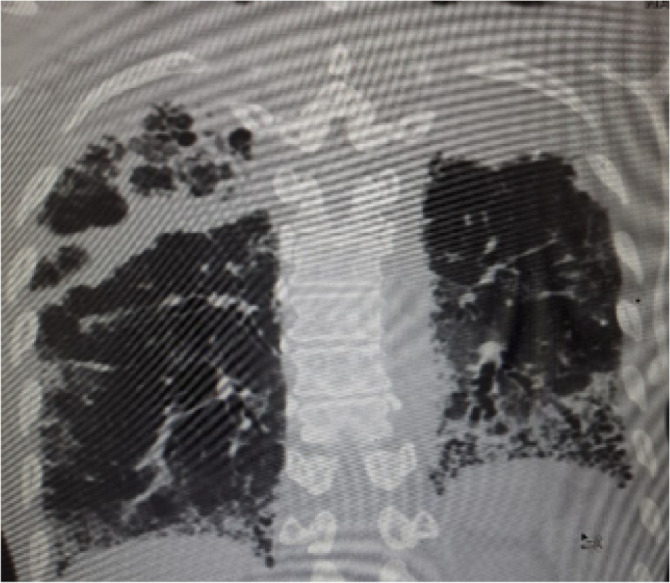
HRCT of chest (Coronal section) showing extensive bilateral basal honeycombing and traction bronchiectasis.
